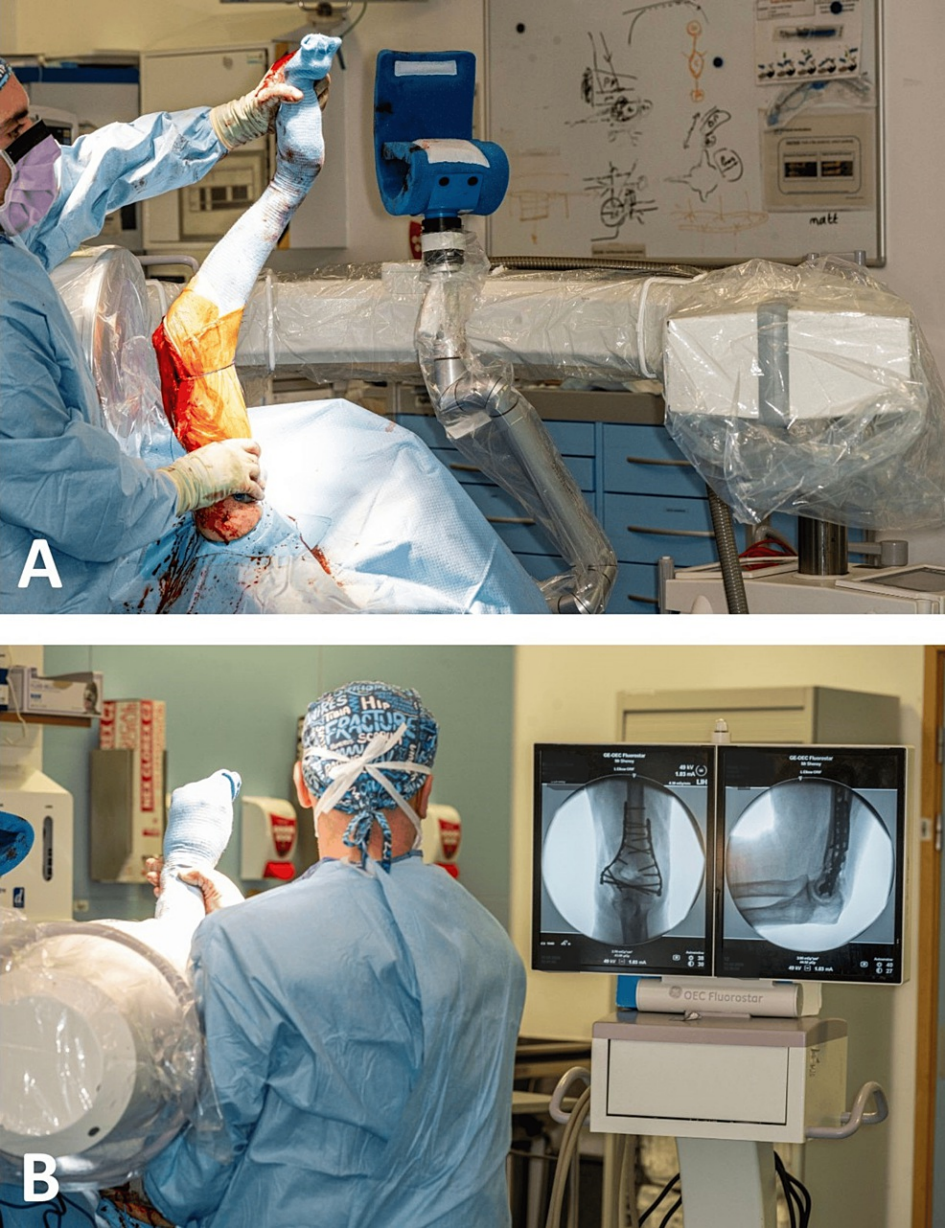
Image demonstrating an anteroposterior radiograph of the elbow. A: The surgeon removes the arm out of the arm holder and internally rotates the shoulder. B: This position allows for a high-quality anteroposterior radiograph of the elbow or distal humerus.
